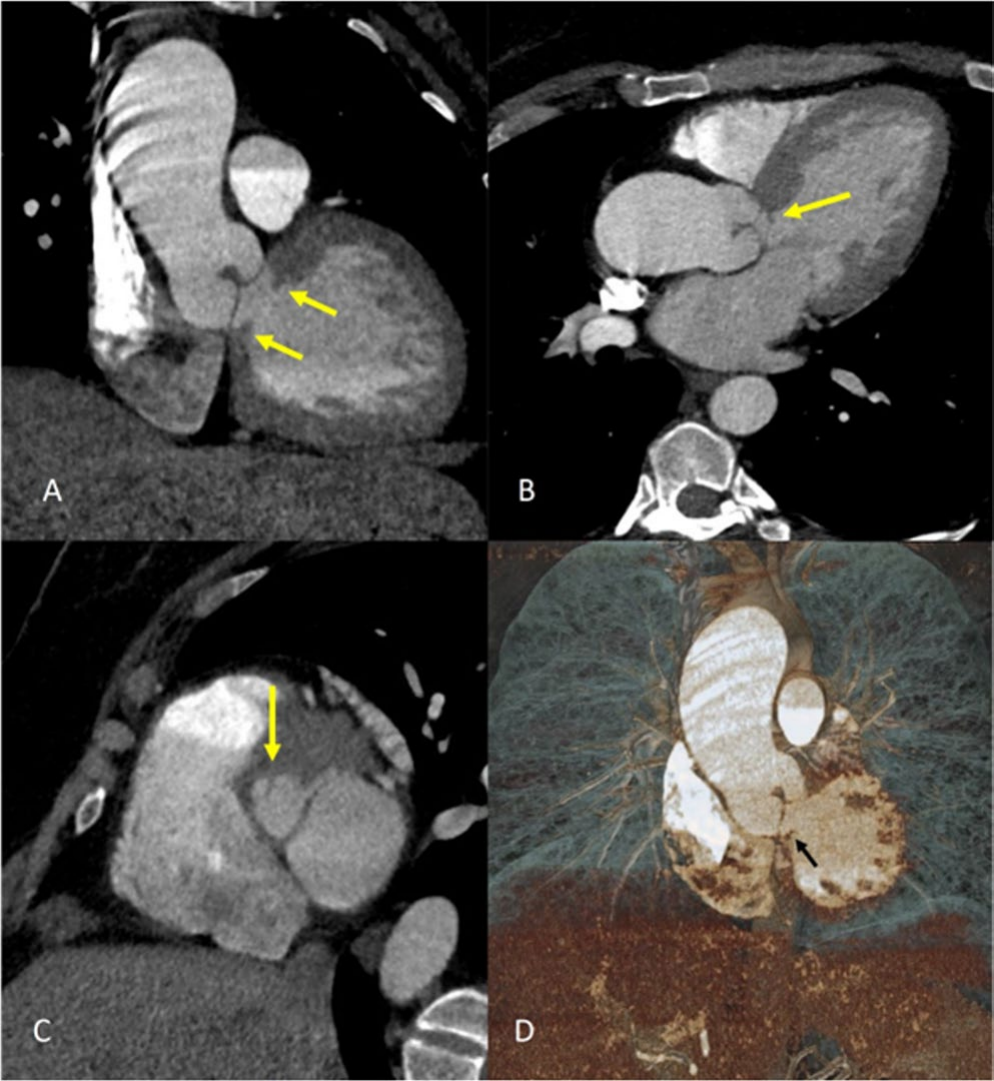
Computed Tomography Angiography Multiplanar Reconstruction Images (A-C) showing linear hypodensity (yellow arrows) in the left ventricular outflow suggesting subaortic membrane. D) Cinematic Volume Rendered Computed Tomography image showing subaortic membrane (black arrow)
